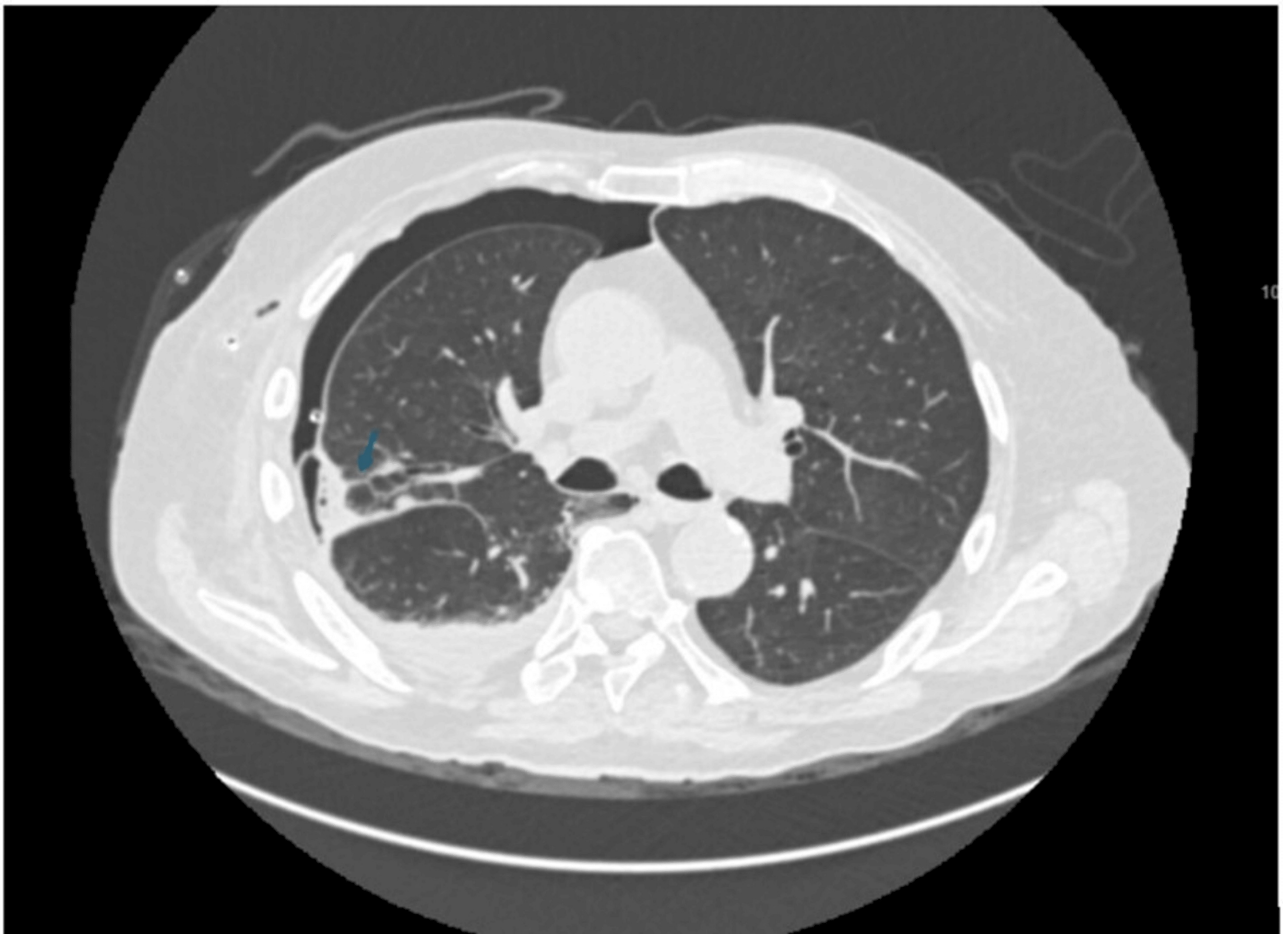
CT scan done five days post chest drain insertion showing possible bronchopleural fistula (BPF) arising from right upper lobe posterior segment bronchiole (marked by arrow)
